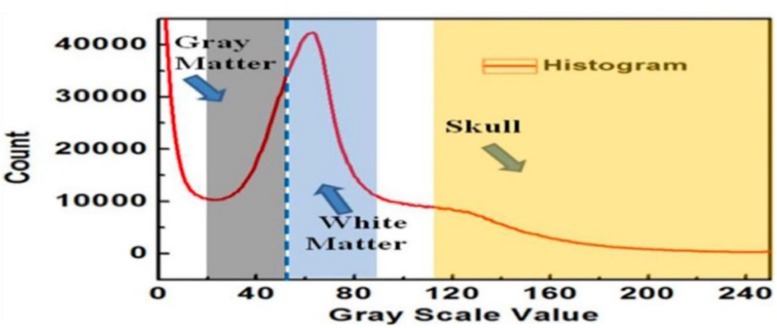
The histogram of the MRI image stack and different window functions applied to separate gray matter, white matter, and skull.
